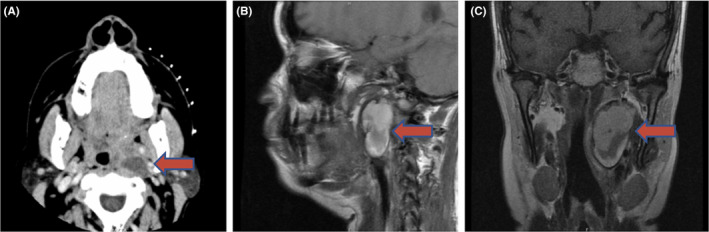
A, Transverse CT demonstrating an ovoid heterogeneous mass with partial occlusion of the oropharynx. B, Sagittal T2‐weighted magnetic resonance scan showing the anterior displacement of the carotid arteries due to the mass effect. C, Coronal T1‐weighted magnetic resonance scan revealing the location of the mass and the effect on surrounding structures
